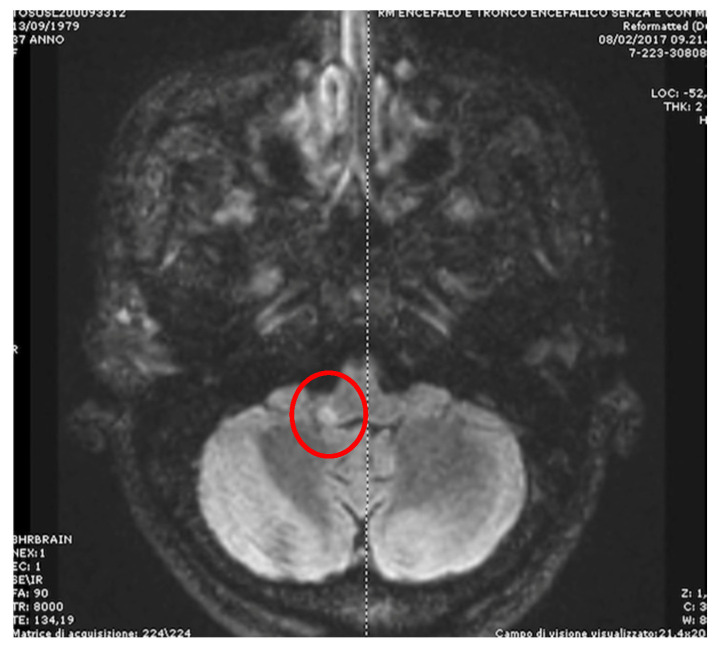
A female, 46 years old, complaining of acute spinning vertigo with no other accompanying symptom, presented to the emergency room: The bedside examination reveals pure torsional nystagmus (see Supplementary Material); MRI (T2-weighted axial images with contrast) shows a demyelinating focal disorder localized in the right posterior medulla at the level of the inferior cerebellar peduncle (red circle).
